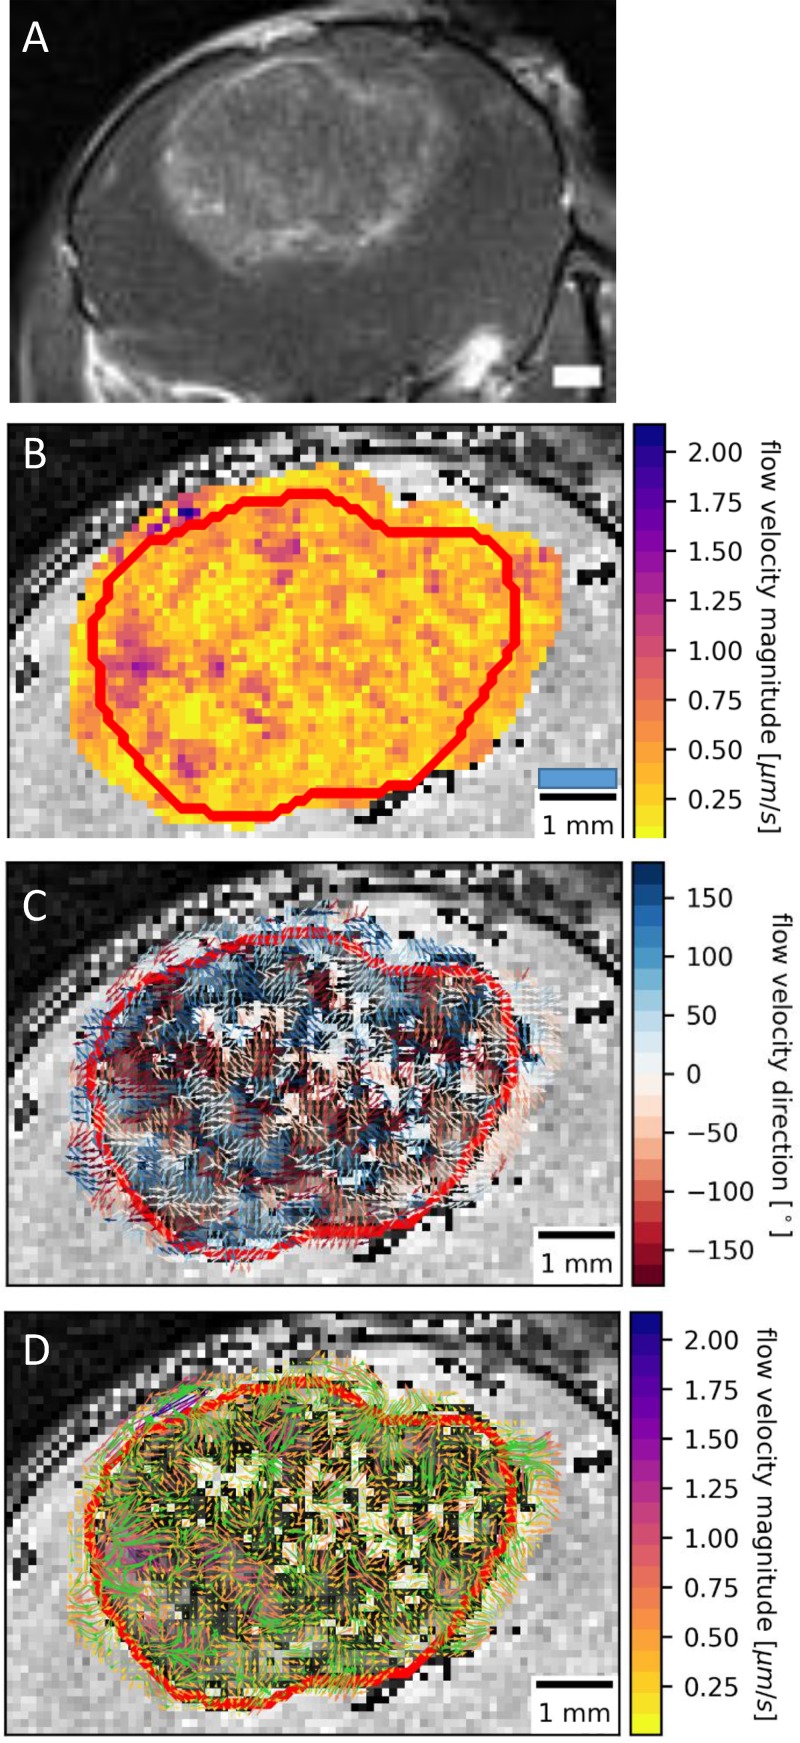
In vivo* heterogeneity of interstitial fluid flow in glioblastoma. (a) T1-weighted MRI with contrast used for identification of tumor analysis region, scale bar = 1 mm. (b) Velocity magnitude output, (c) velocity vector output, and (d) velocity magnitude map overlaid with velocity vector plots from computational analysis in the selected region with the tumor border shown in red.
